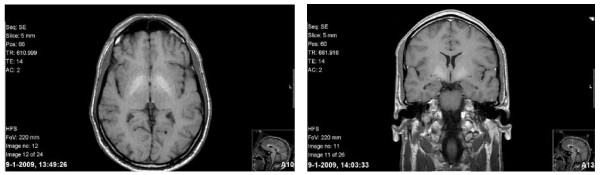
MRI scan of the brain of our 49-year-old patient exposed to Manganese. Bilateral high T1 signal intensity in the globus pallidus is shown. Left: transversal view; right: coronal view.
